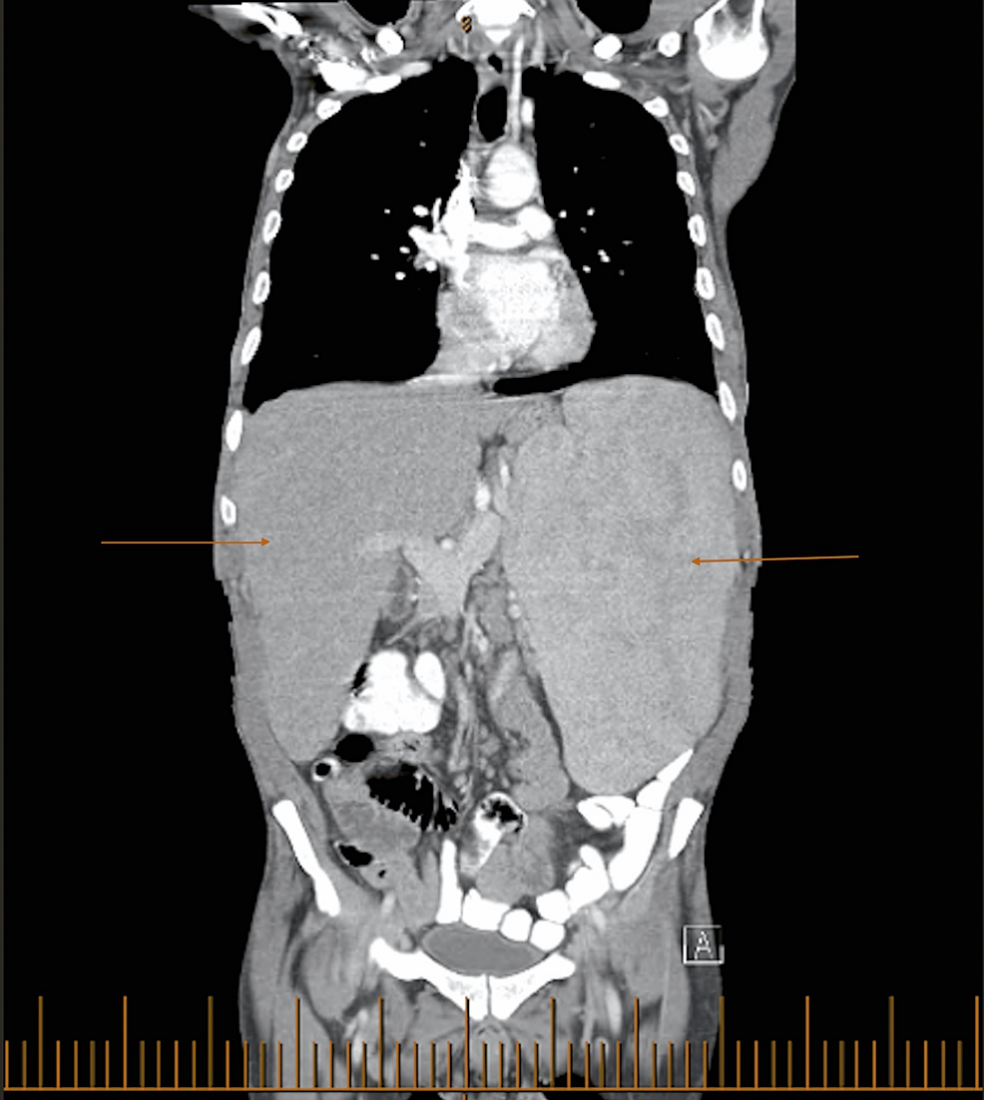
CT abdomen and pelvis without contrast revealing hepatosplenomegaly
